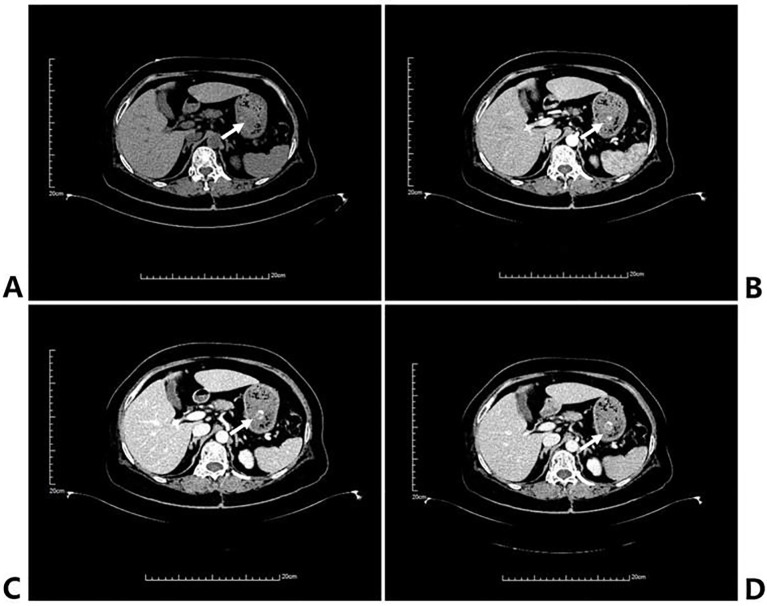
The manifestations of CT scans in different stages of the metastatic tumors in the stomach. (A) CT plain scan shows a locally nodular protrusion in the gastric body wall, appearing as a slightly hypodense shadow. (B–D) The arterial, portal venous, and delayed phases demonstrate heterogeneous, significant nodular enhancement.
